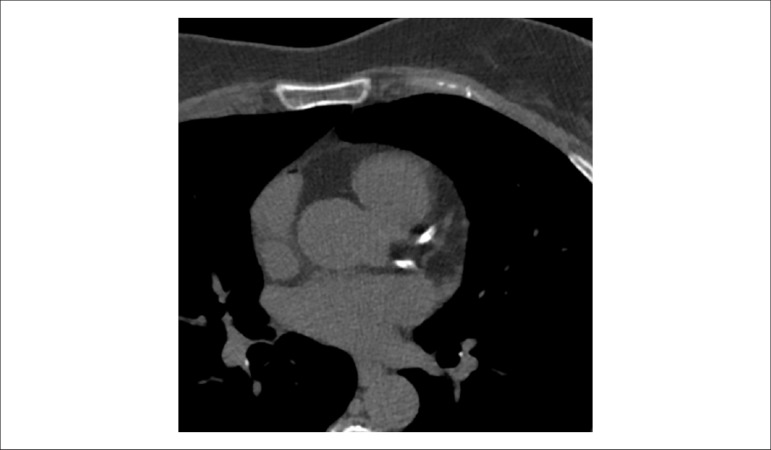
Case 5 - Angio-CT revealing severe calcification in coronary arteries.
